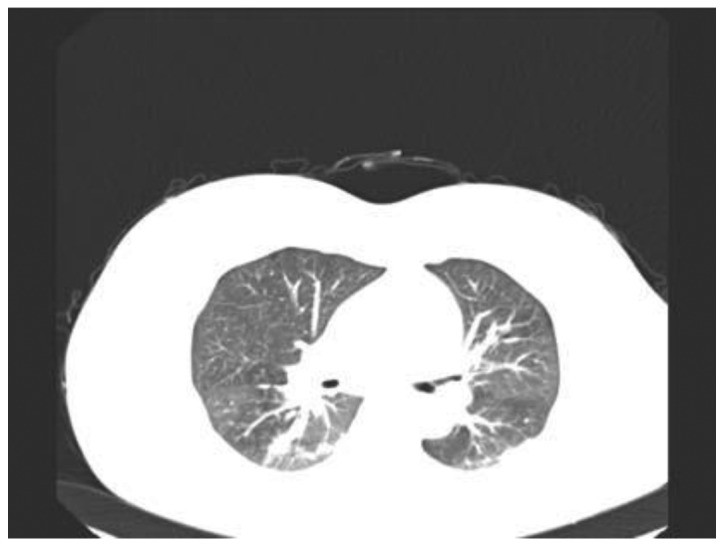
Lung computed tomography (CT) revealed interstitial pneumonitis in Case 1.
